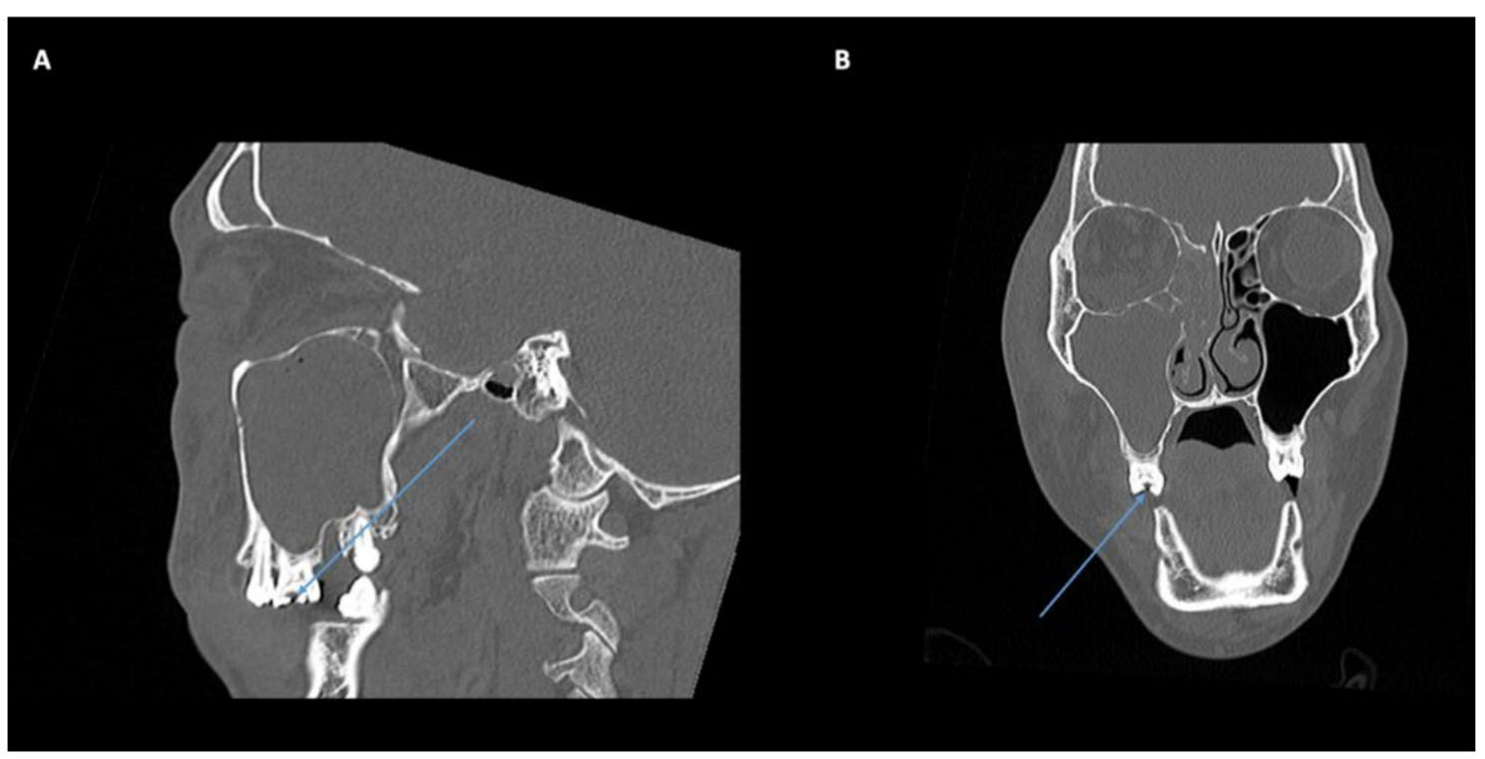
Case 2: The odontogenic source of infection is demonstrated on the CT facial bones imaging. A-B: sagittal and coronal CT images demonstrating hypoattenuation of the upper right molar (16) occlusal, and secondary opacification of right maxillary sinus
